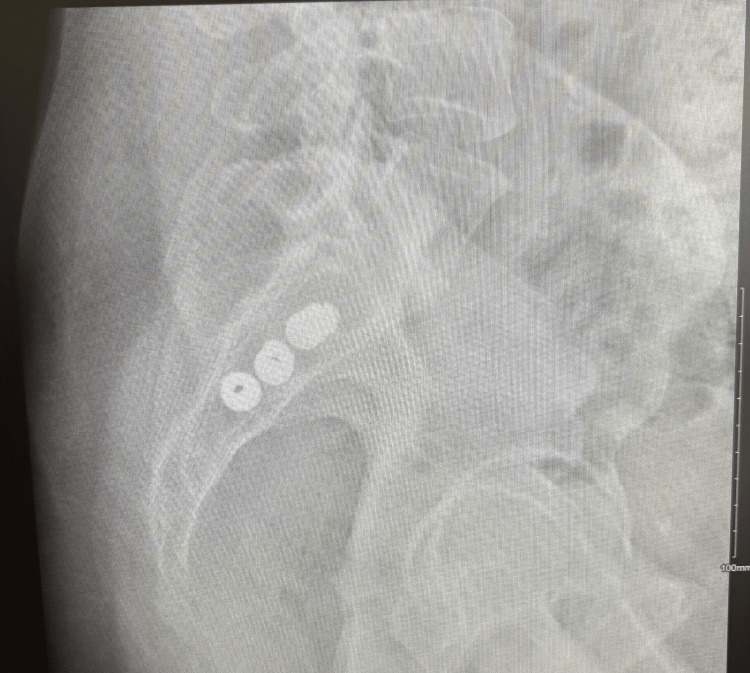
Postoperative Lateral Radiograph Postoperative lateral view showing stable implant positioning at six months postoperatively. No signs of hardware loosening, subsidence, or migration are observed.
